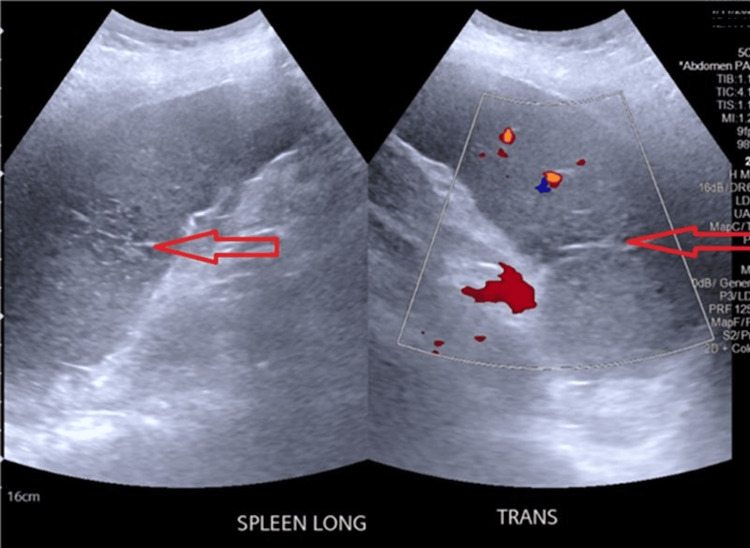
Ultrasound of the spleen demonstrating features of splenic infarction Ultrasound image of the spleen showing a focal, wedge-shaped hypoechoic region centrally, without detectable color or spectral Doppler flow, findings consistent with splenic infarction. The splenic hilar vessels are patent, and there is no perisplenic fluid collection. The ultrasound was used to confirm the CT findings and to exclude other structural abnormalities or abscess formation. The spleen is enlarged, measuring 15.7 cm in length.
